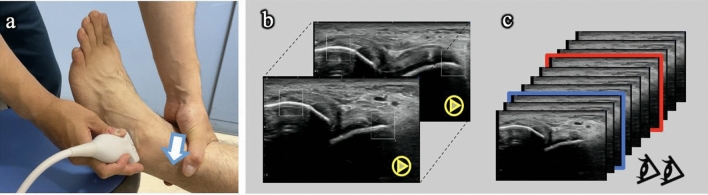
Sonographic data collection and measurement methods. (a) Reverse anterior drawer test from non-stress to loading conditions of the tibia posterior (arrow), viewed along the ATFL long axis. (b) ALMS; Sonographic video with one amplitude of joint motion cut/selected offline, followed by attachment of the regions of interest (ROI)s to two points on top of the talofibular joint to detect the trajectory. (c) Manual measurement; The two testers measure the joint distance individually by converting static images and judging minimal (blue) and maximal (red) distances. *ALMS* automated length measurement system, *ATFL* anterior talofibular ligament.
